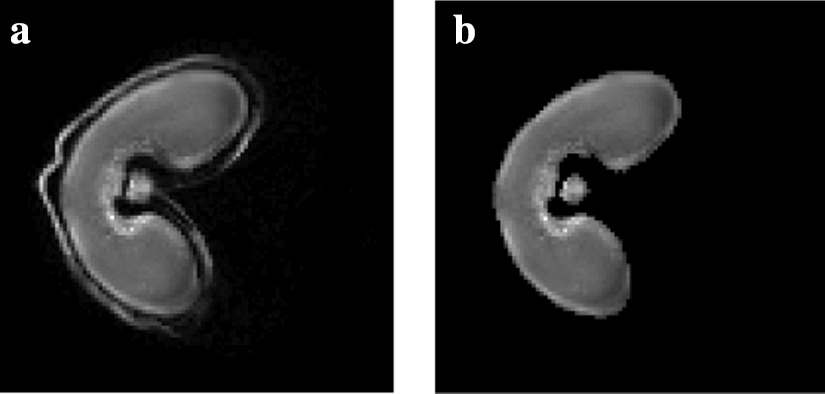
Segmentation of MRI images. a Original MRI image and b resulting segmented image. The pericarp (outer structure) of the wheat grain is removed in the segmented grain so as to compare MRI and MALDI–MS images more easily
